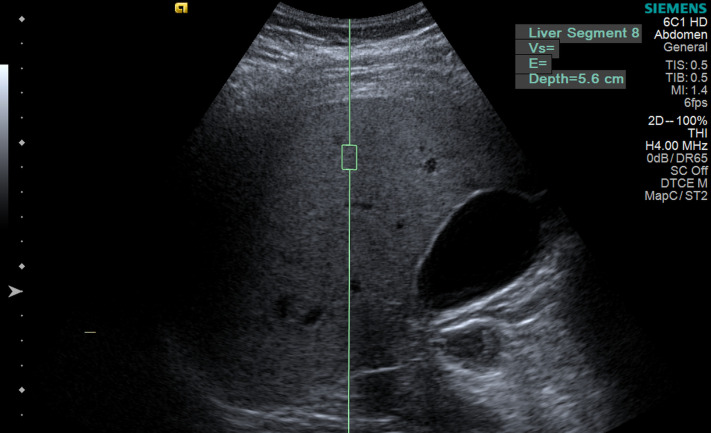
Ultrasound elastography measurement of liver stiffness. Author’s source.
